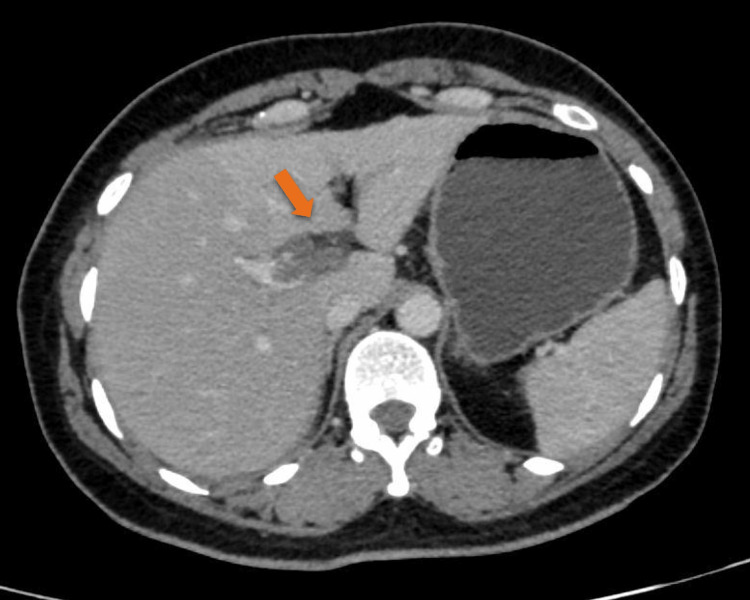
Abdominal CT scan showing thrombosis at the bifurcation of the main portal vein extending to the left branch.
